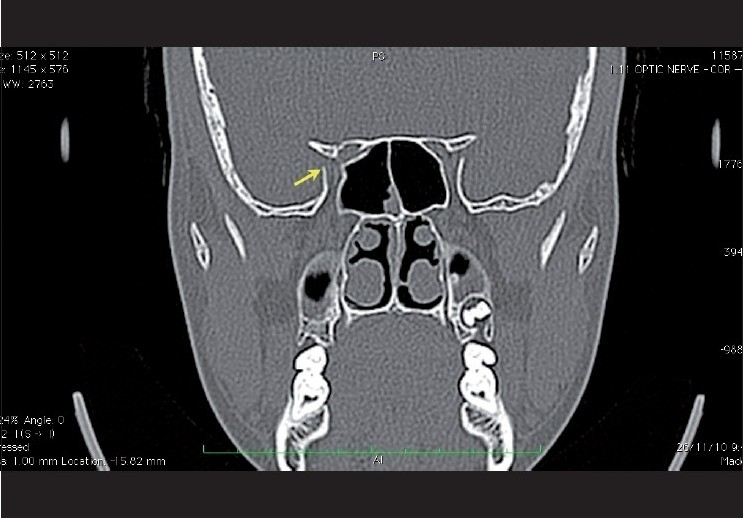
Coronal CT scan of the orbit at 1mm interval for the optic canals in the bone window setting showing a fracture of the medial wall and the roof of the right optic canal (Yellow arrow)
